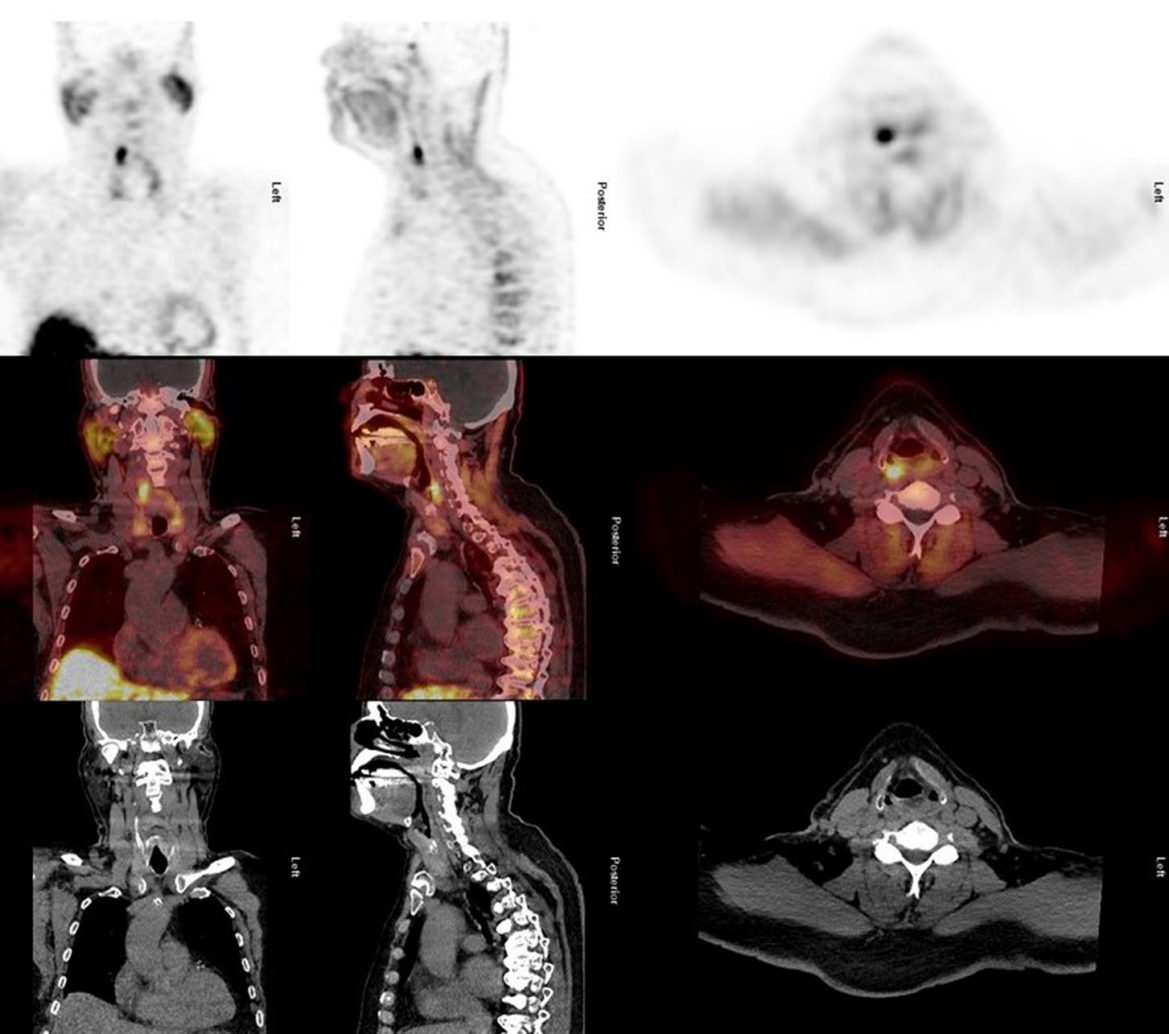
Upper panel: [^18^F]fluorocholine PET images at the level of the undescended PTA. Middle panel: overlay of the [^18^F]fluorocholine PET and high-dose CT images at the level of the undescended PTA. Lower panel: high-dose CT images at the level of the undescended PTA. Left, middle and right columns: coronal, sagittal and transversal views, respectively
